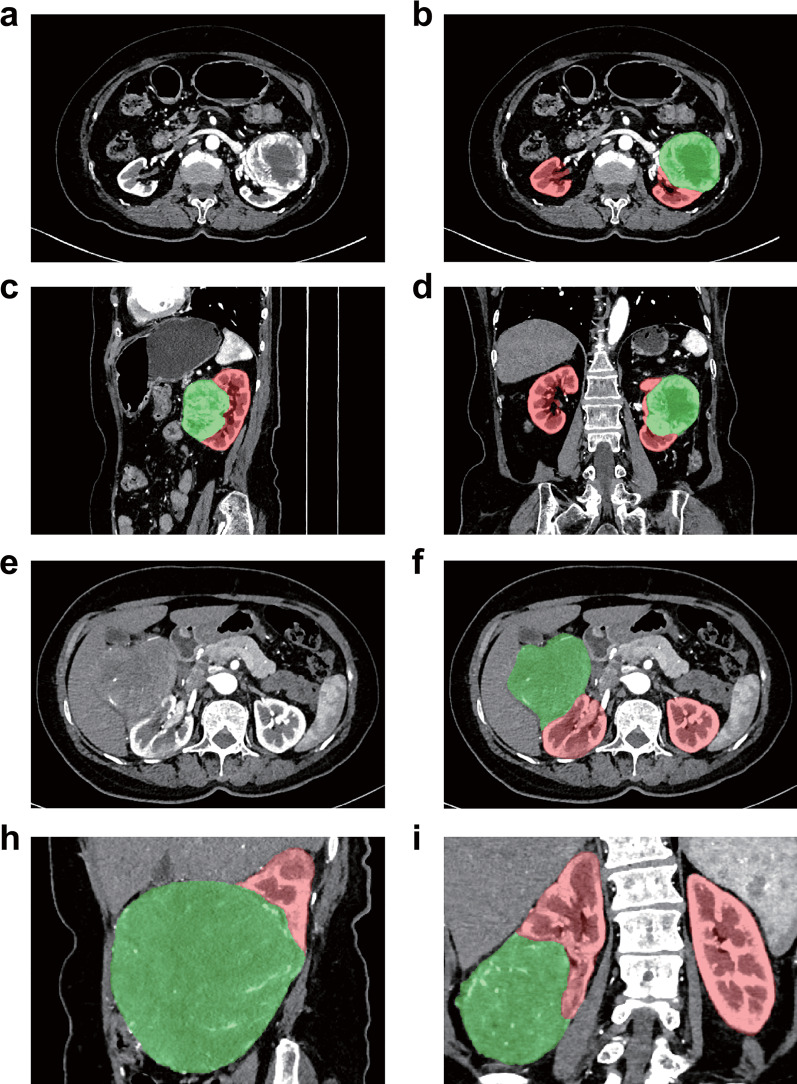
CT images after automatic segmentation by 3D UNET in external dataset. a–d Male, 77, clean cell renal cell carcinoma, underwent radical nephrectomy, high Ki67 (10%). e–i Male, 60, chromophobe renal cell carcinoma, underwent radical nephrectomy, low Ki67 (3%)
